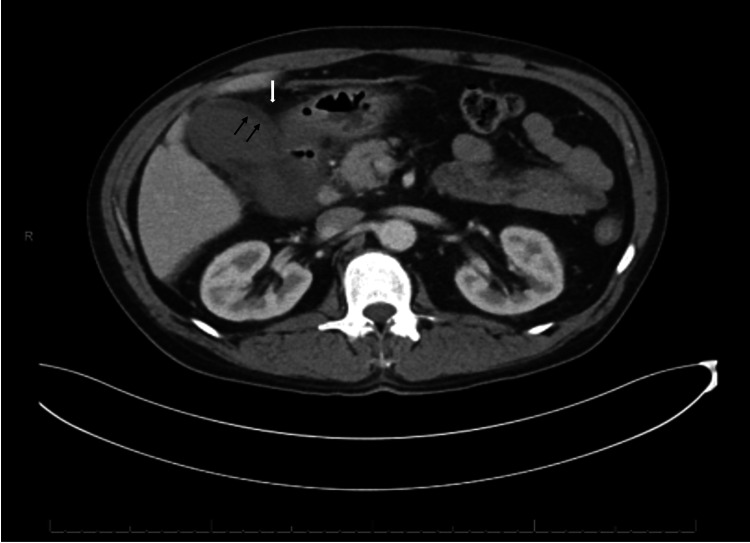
CT of the abdomen and pelvis with contrast, demonstrating significant inflammatory stranding (white arrow), gallbladder wall thickening (black arrows), and an overall loss of enhancement of the gallbladder wall, with associated distention concerning for acute cholecystitis with a possible gangrenous component. CT, computed tomography
